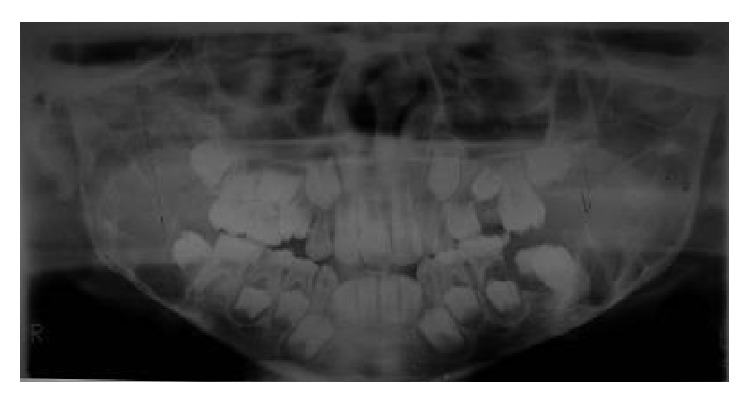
Orthopantomogram showing bilateral multilocular radiolucencies involving posterior body, ramus of mandible, and condyle typically spared.
